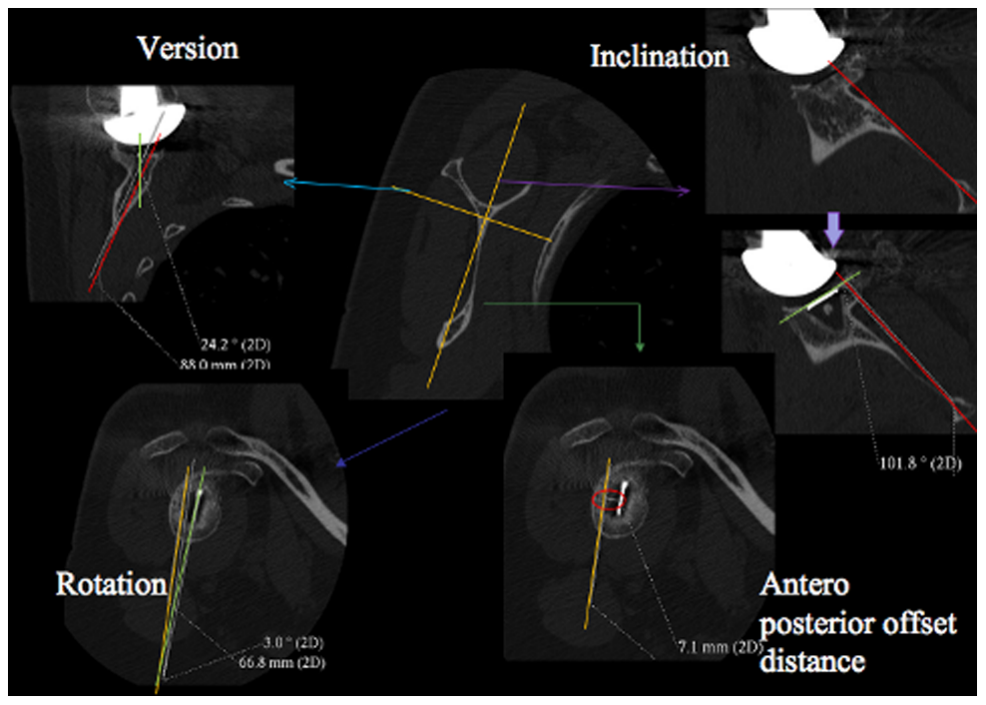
Definition of the four glenoid component positioning parameters. : version (top left) is the angle between the green line (i.e. the orientation of the glenoid component surface) and the red line (i.e. the orientation of the scapula plane); inclination (top right) was measured from the orientation of green line (i.e. the superior-inferior line of the glenoid component) and the red line (i.e. the orientation of the supraspinatus fossa line (SFL)); rotation (bottom left) is the angle between the orange line (i.e. the orientation of the scapula plane) and the green line (i.e. the superior-inferior line of the glenoid component); off-set distance(bottom right) was measured as the shortest distance between the white marker in the CT image (i.e. the centre of the glenoid) and the orange line (i.e. the orientation of the scapula plane). The orange lines in the middle image indicate the orientation of the scapula blade used.
